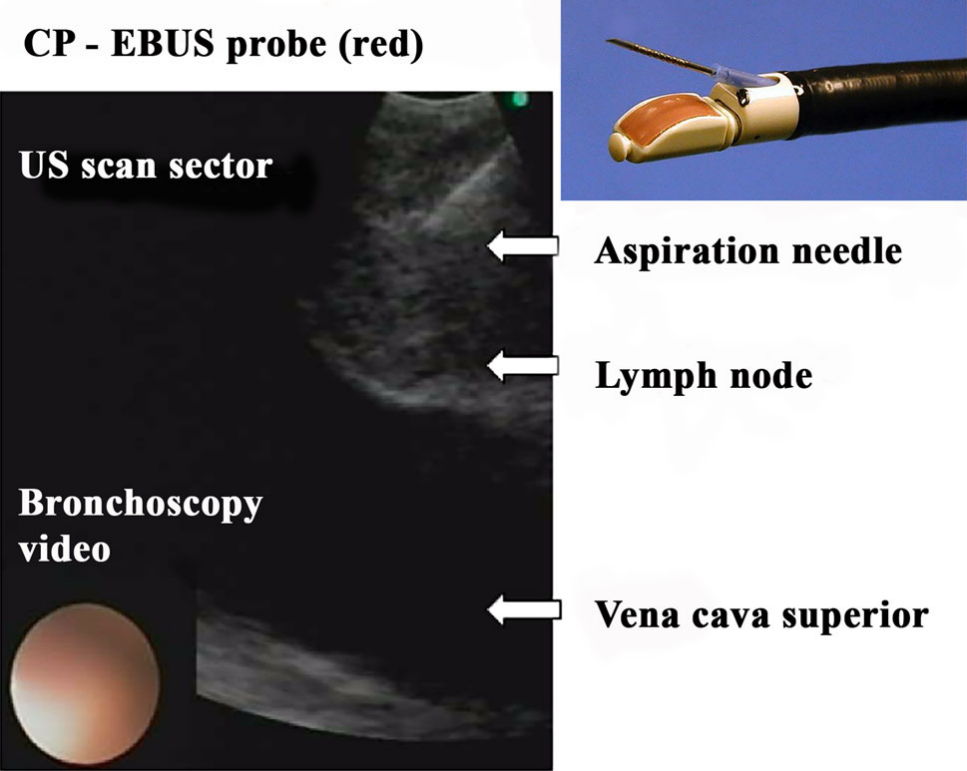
Convex probe endobronchial ultrasound (CP-EBUS) guiding real-time transbronchial fine-needle aspiration of a mediastinal lymph node. The sampling needle is visualized sonographically inside the lymph node. Video bronchoscopy is displayed simultaneously (*bottom left*). The transbronchial needle system emerges from the bronchoscope’s working channel just proximal to the CP-EBUS probe (*top right*)
